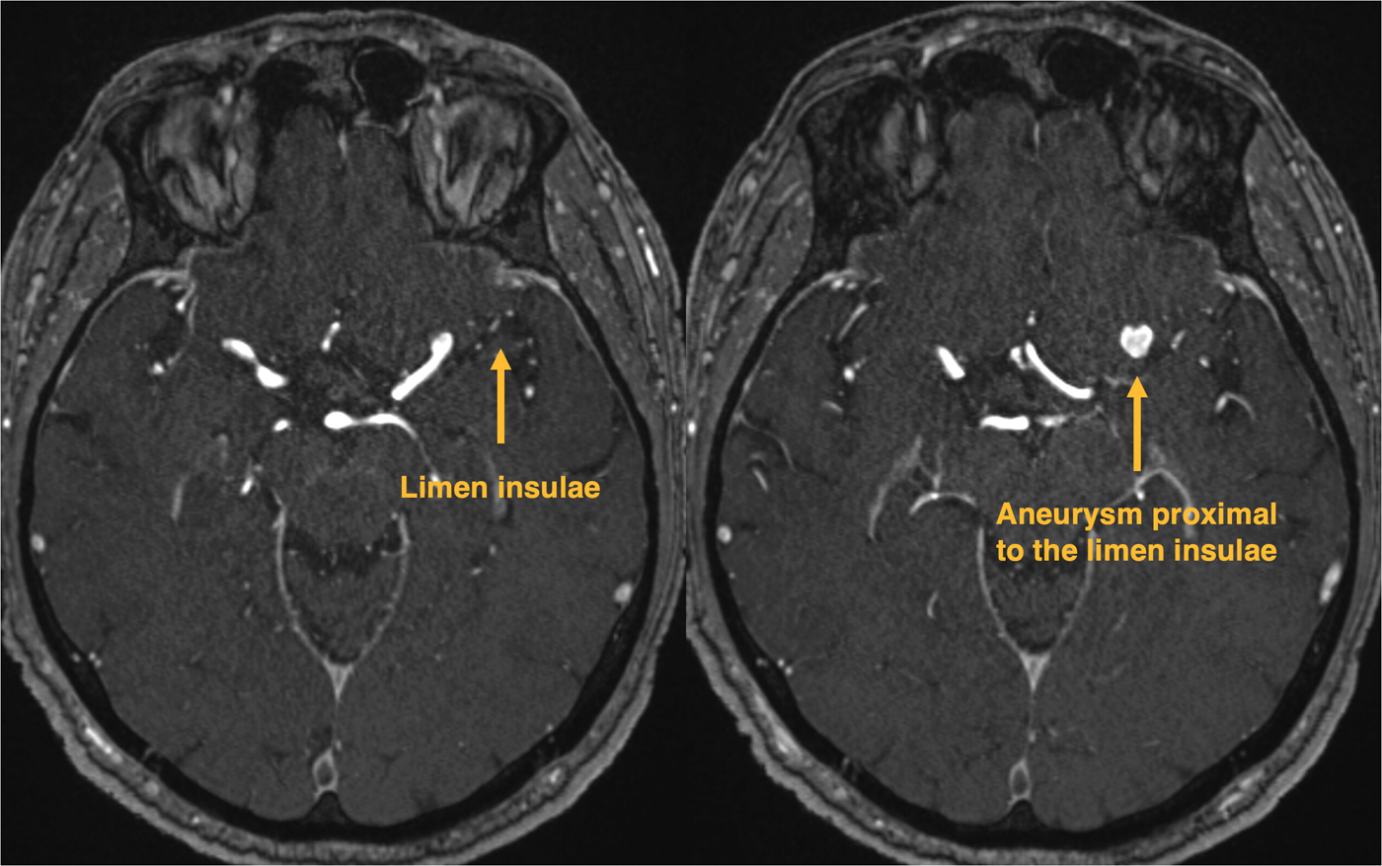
Minipterional case. Native time of flight angiography MRI with a left-sided MCA aneurysm, located proximally to the limen insulae.
